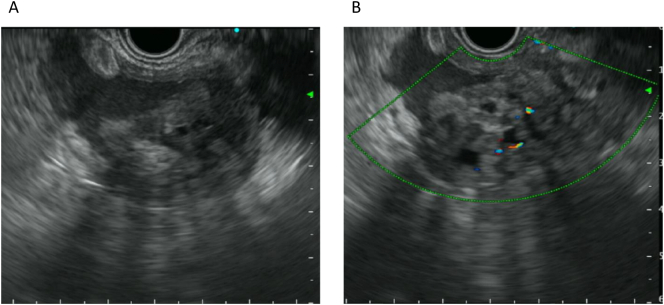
A; EUS, B; EUS with collor doppler imaging. EUS showed that the nodule was protruding into the lumen and had blood flow.
